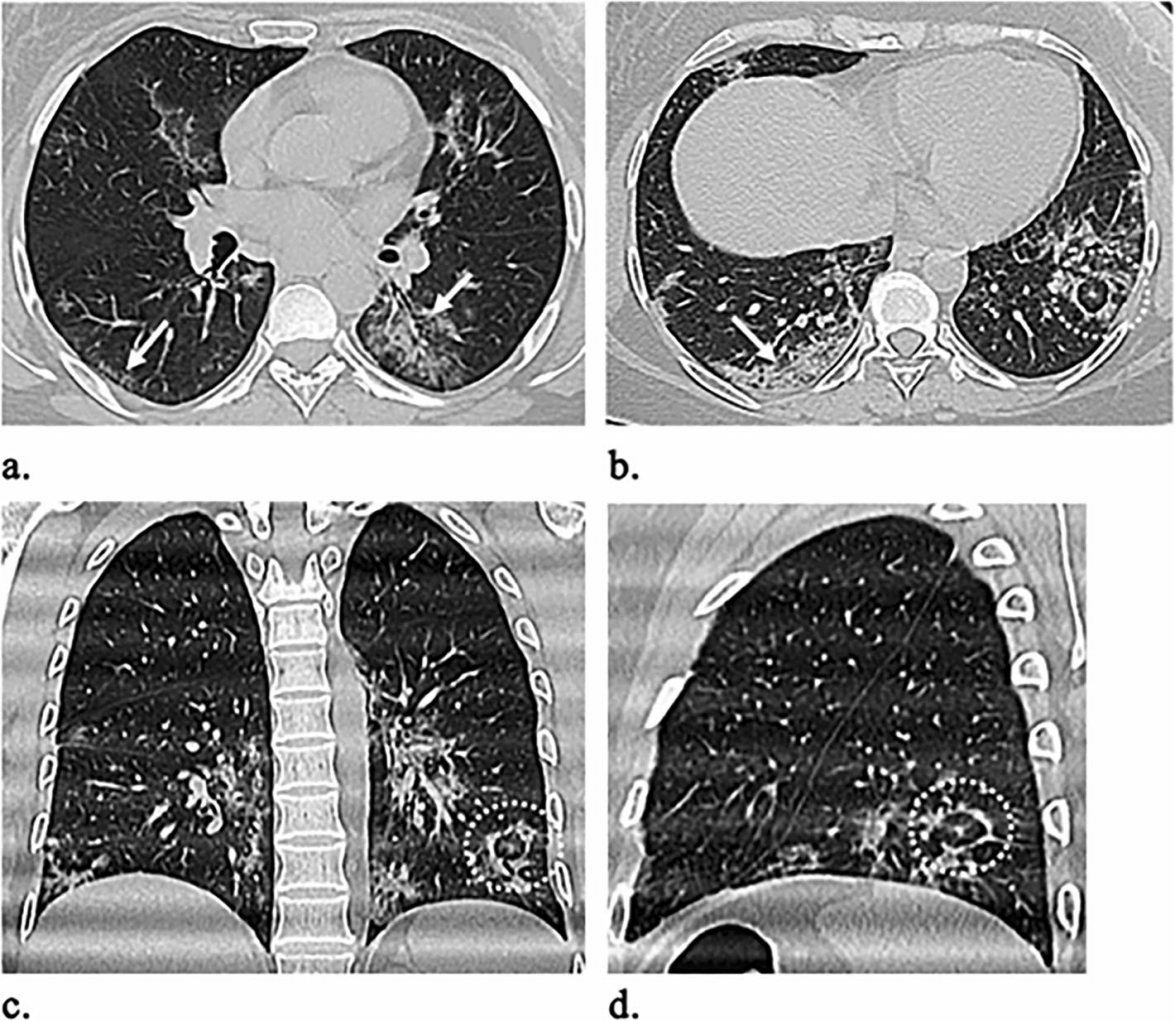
A 42-year-old female patient experienced high-grade fever and cough for 12 days. O_2_ saturation was 95%. a, b Axial, c coronal, and d sagittal unenhanced HRCT images reveal bilateral multifocal subpleural lower lobe predominant GGO and crazy paving (white arrows) with left lower lobe reversed Halo sign (dashed circle)
